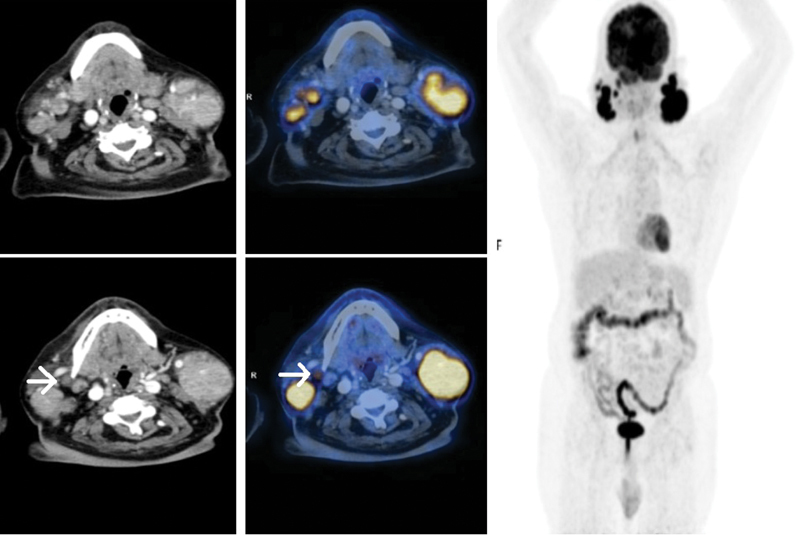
Axial section on computed tomography (CT), positron emission tomography (PET), and fused PET-CT showing intense fluorodeoxyglucose (FDG) uptake in the parotid region corresponding to multiple enhancing nodules in both lobes of parotid gland on axial CT (
